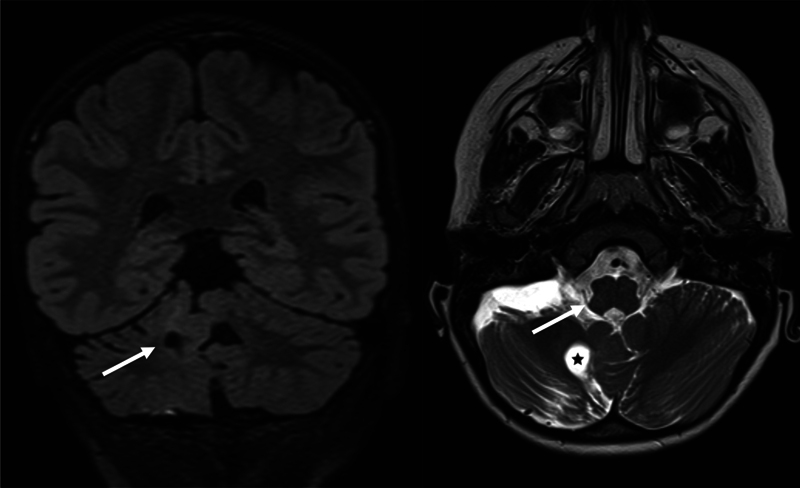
Brain MRI. (Left) Coronal FLAIR shows the extension of pathological tissue to the right dentate nucleus (arrow), apex of Mollaret's triangle. (Right) Axial T2. The bulb shows normal morphology and signal. The surgical cavity (star) is distant from the course of the accessory nerve (arrow).
